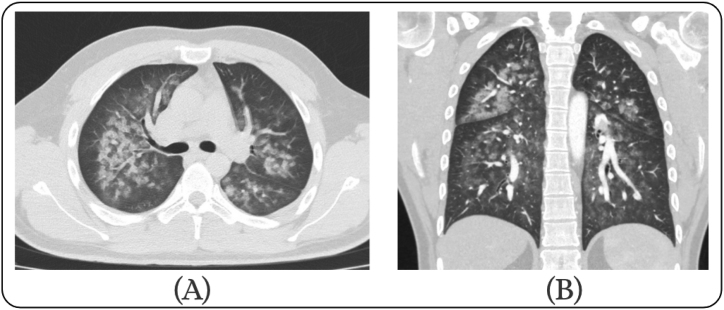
Axial CT [A] and Coronal CT [B] images show diffuse bilateral nodular opacities and interstitial thickening, consistent with diffuse alveolar hemorrhage.
